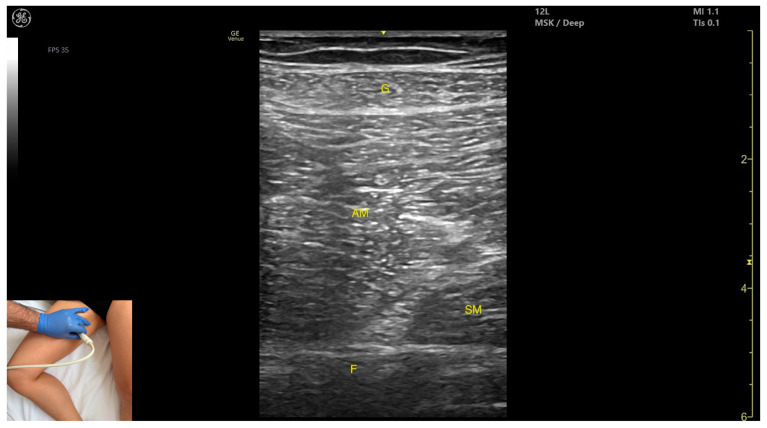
Ultrasound anatomy of adductor magnus (AM) with key landmarks: G—*gracilis*; AM—*adductor magnus*; SM—*semimembranosus*; F—femur.
